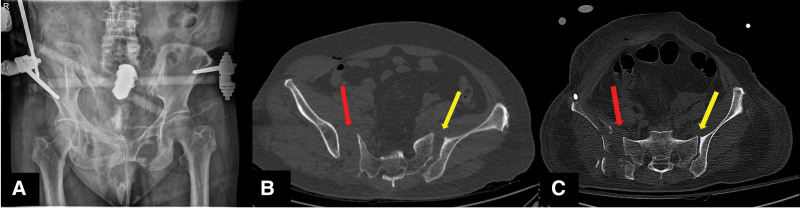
Damage control external fixation. (A) Pelvis AP x-ray demonstrating volume reduction of the pelvic cavity (B) axial view of computed tomography demonstrating anatomic reduction of the left SI joint (yellow arrow), still widening of the right SI joint (red arrow) compared with (C) preoperative image. AP = anteroposterior, SI = sacroiliac.
